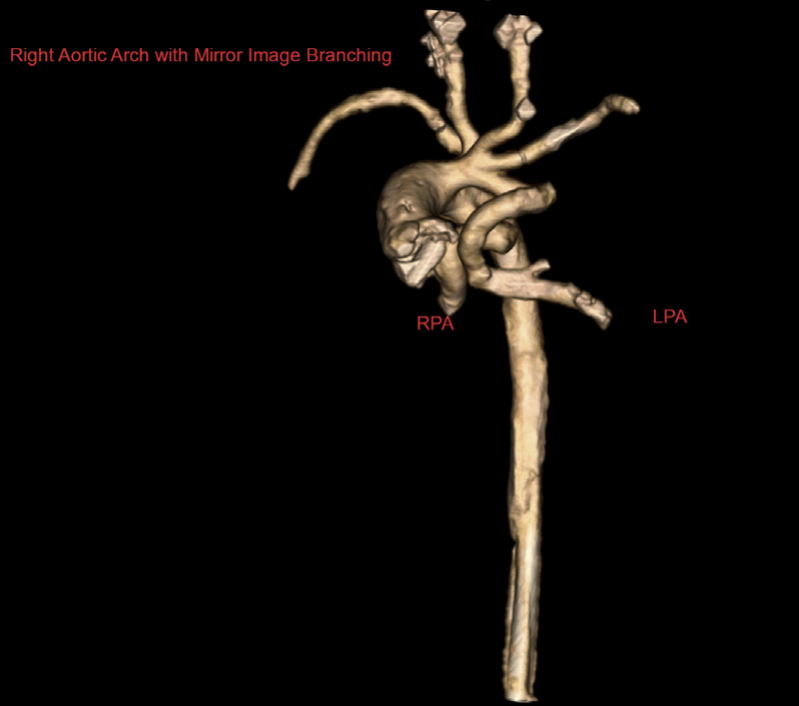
Volume-Rendered Computed Tomographic Reconstruction Showing a Right Aortic Arch With Mirror Image Branching and Discontinuous Pulmonary Arteries The left pulmonary artery (LPA) is seen originating from the undersurface of the left innominate artery. RPA = right pulmonary artery.
